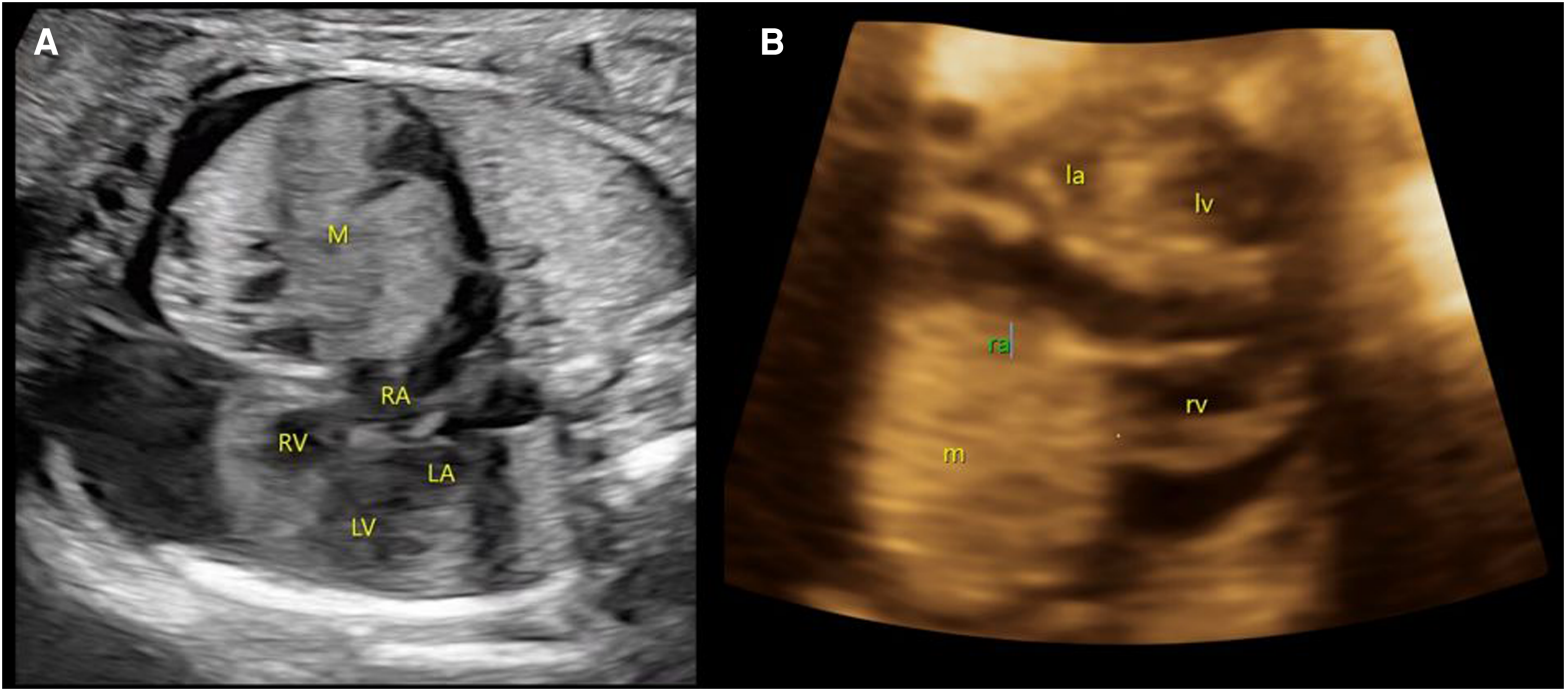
Prenatal ultrasound images. (A) At 26 weeks of gestation, a mixed mass (M) in the pericardium of the fetus with size approximately 27 × 26 mm with pericardial effusion is demonstrated. (B) At 37 weeks of gestation, a slightly strong echo mass (m) can be seen in the right pericardial cavity, with a range of approximately 37 × 36 mm. M/m, mass; RA/ra, right atrium; RV/rv, right ventricle; LA/La, left atrium; LV/Lv, left ventricle.
